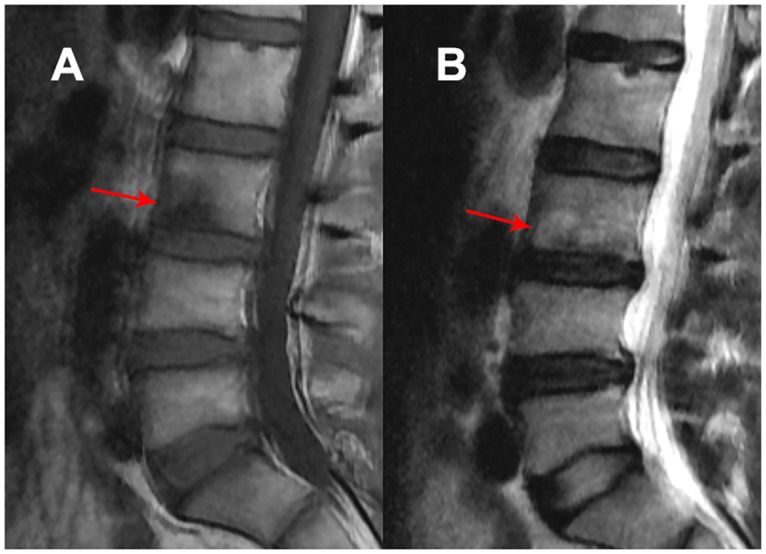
Type I Modic changes in lumbar spine. Hypointense changes in T1-weighted images (A) and hyperintense changes in T2 weighted MRI images (B).
